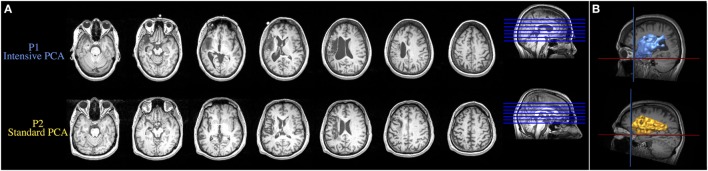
(A) Axial MRI images showing the lesions resulting from a left ischemic stroke in the middle cerebral artery territory. (B) Reconstruction in 3D of the brain lesion of both patients. Upper and lower images are from the patient who received the intensive condition and the patient who received the standard regimen, respectively.
